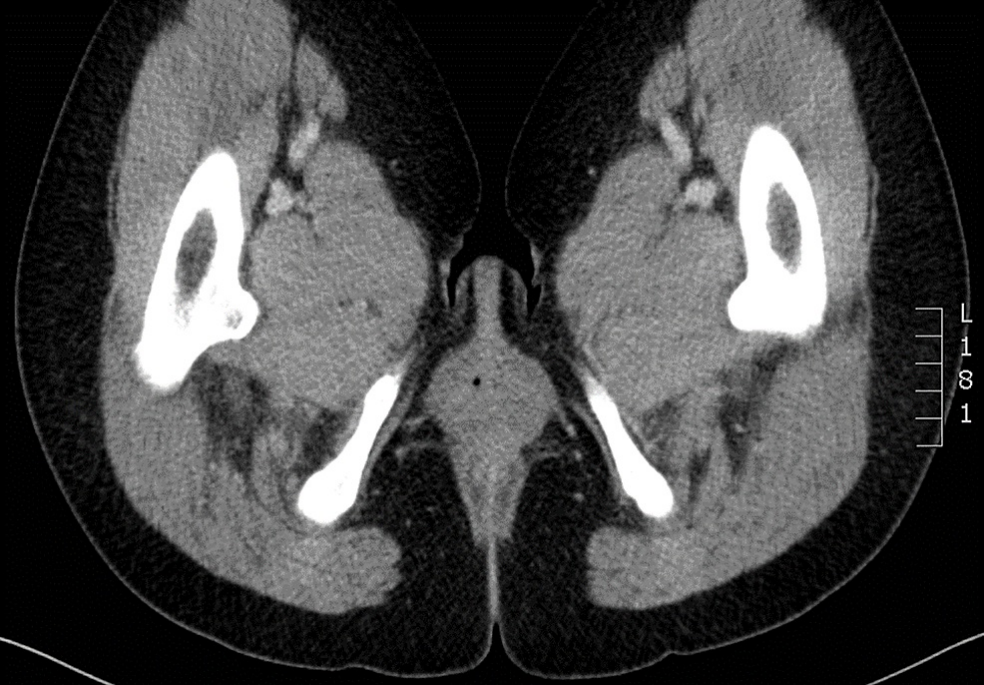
Axial section of a contrasted computed tomography of the abdomen and pelvis, with no acute findings.
